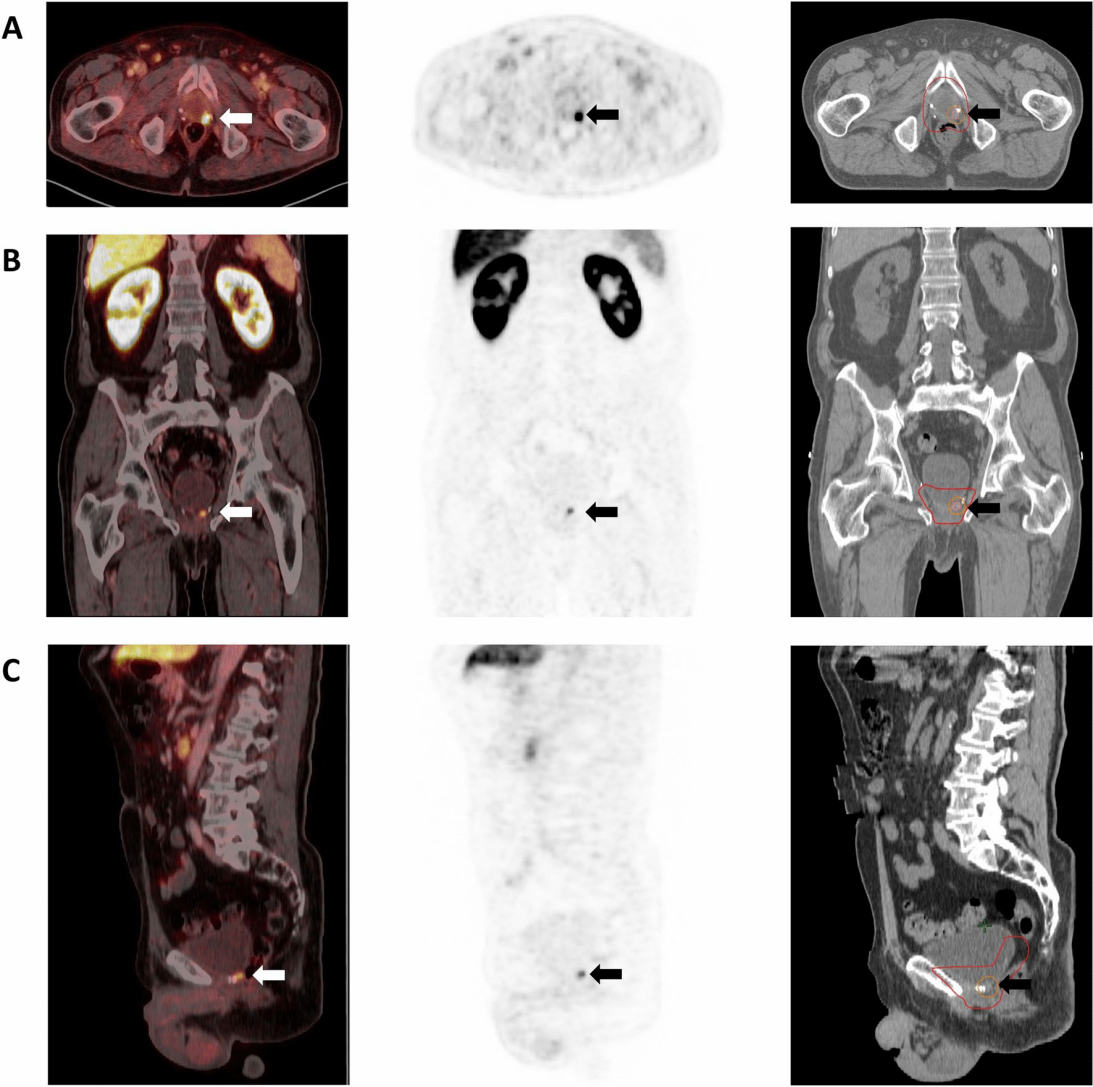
Example of a patient with ^18^F-flotufolastat-guided salvage radiotherapy for local recurrence located caudally to the bladder. The Figure shows an axial (A), coronal (B) and sagittal (C) plane of the PET/CT-fusion, PET sequence, and the resulting radiotherapy contouring (red = planning target volume, orange = simultaneous-integrated boost, pink = gross tumor volume).
